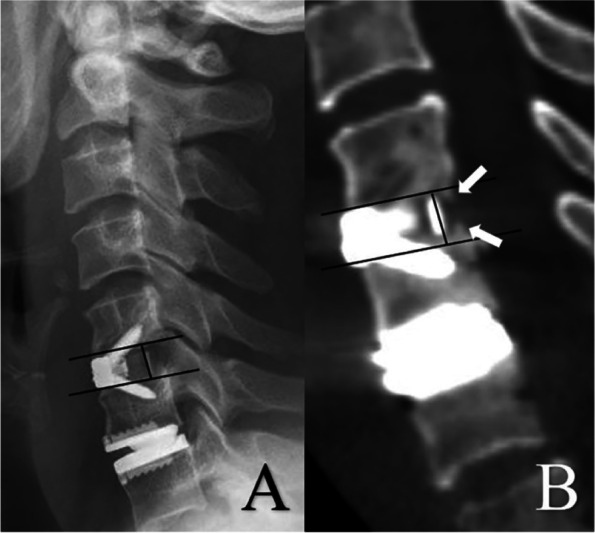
A lateral X-ray at 3-month follow-up was used to confirm the shape of endplates and the location of the marker line (A). The height of bone tissue along the posterior border of the cage was measured in the sagittal CT scan as the reflection of early fusion effect. (The white arrows in B)
